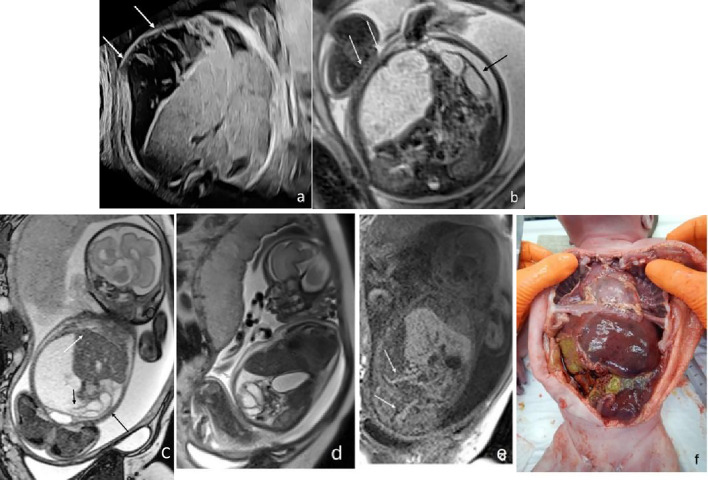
Meconium peritonitis following intestinal volvulus and perforation. Axial US of the upper abdomen (a) and axial (b), coronal (c), and sagittal (d) T2-W MR images at 30GW show voluminous ascites with calcifications and multiple septations (white arrows, b) causing significant hepatic distortion. Note multiple distended, fluid-filled bowel loops in the left hemiabdomen (black arrows, b, c), with suspected bowel perforation (c, short black arrow). Coronal T1-W image (e) reveals microcolon and rectum (white arrows, e), reduced meconium volume, and anomalous meconium signal for gestational age. Note also diaphragmatic elevation and severe bilateral lung hypoplasia (white arrow, c). Autopsy after *in utero* fetal demise revealed a conglomerate of necrotic intestinal loops, entangled and encased in a fibrous meconium plastron secondary to volvulus and perforation (f)
